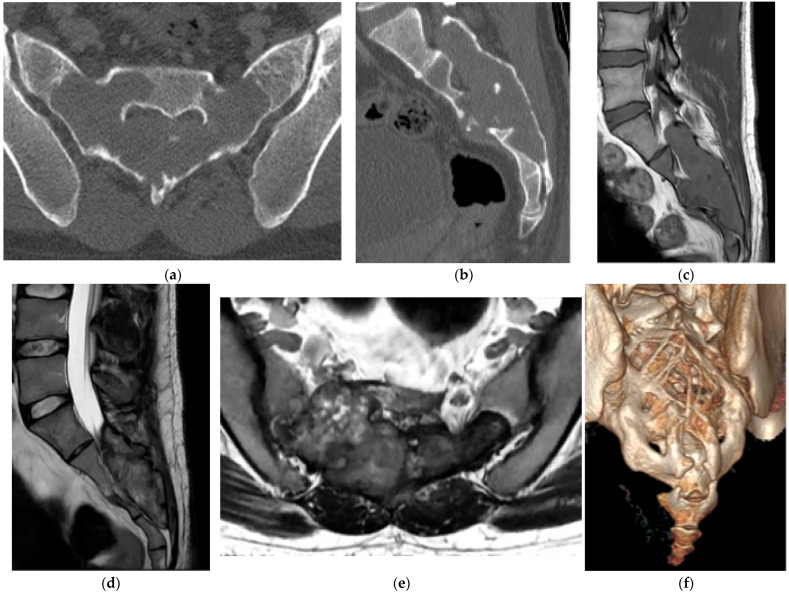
Chondroblastoma. (a) Axial and (b) sagittal CT bone window, expansile and lytic mass centered in the sacrum. No matrix calcification is present in this case. There is severe narrowing of the sacral canal. (c) Sagittal T1 MR: the mass is isointense to the paraspinal muscles. (d) Sagittal T2 MR: there is heterogeneously high T2 signal representing chondroid elements. (e) Axial T2 MR: the mass is expansile and causes severe narrowing of the sacral canal. (f) Volume-rendered reconstruction shows the severely thinned and remodeled cortex of the posterior sacrum.
